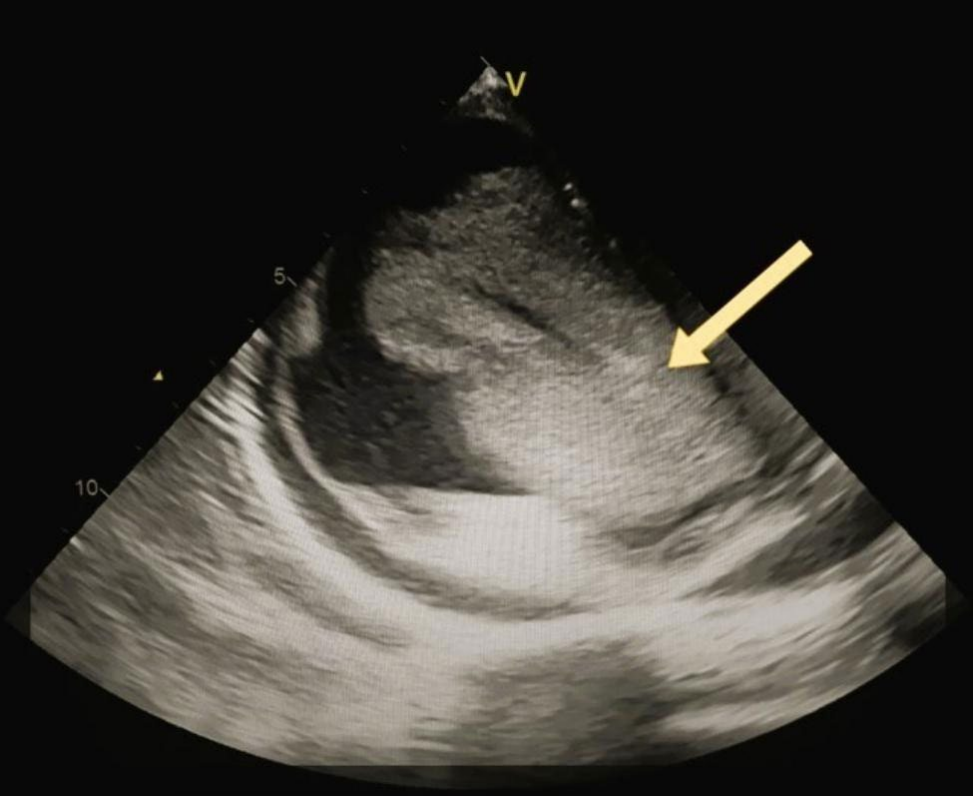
An esophageal view by the trans-thoracic echocardiogram which represents a large atrial thrombus extended to the right ventricle crossing the tricuspid valve
